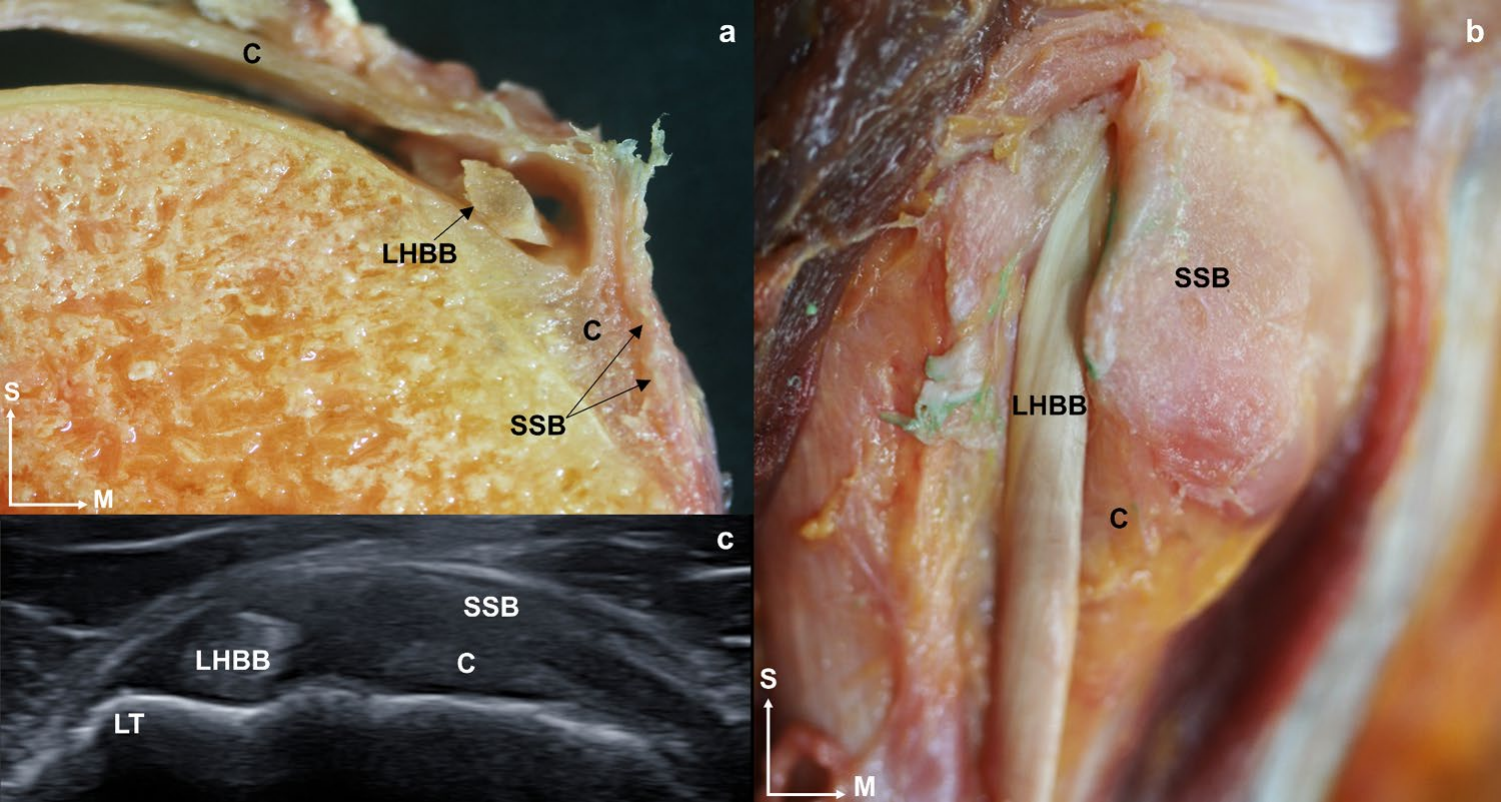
a A sagittal sectional cut of the humerus where it is possible to see the long head of the biceps brachii tendon inside the capsule and surrounded by the subscapularis tendon. b An anterior view of anatomical dissection where the subscapularis tendon is cut to leave the extracapsular part of the long head of the biceps brachii tendon visible. c An ultrasound image in the transverse axis of the long head of the biceps brachii tendon and in the longitudinal axis of the subscapularis tendon before its insertion into the lesser tubercle of the humerus, and before joining the fascia enveloping the infraspinatus. Abbreviations *LHBB*: long head of the biceps brachii, *C*: capsule, *SSB*: subscapularis, *LT*: lesser tubercle, *ISP*: infraspinatus, *A*: anterior, *M*: medial, *S*: superior
